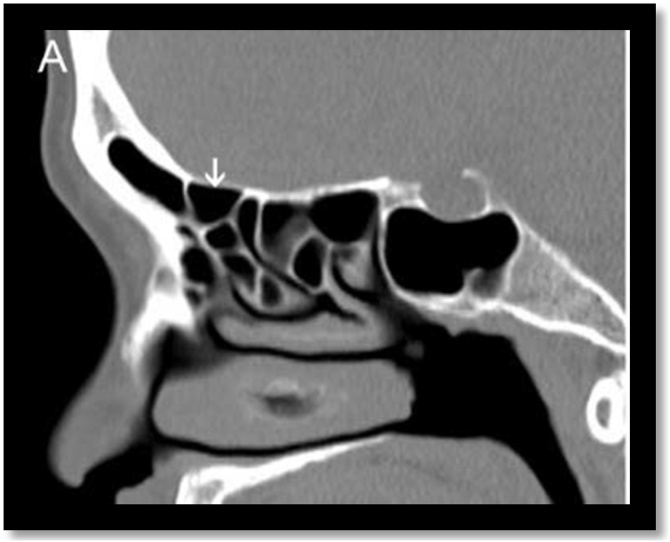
Supra bulla cell. Sagittal computed tomography section demonstrates an example of supra bulla cell (white arrow).
